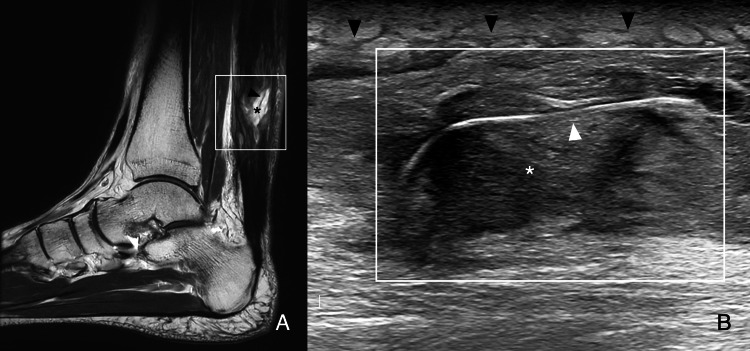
MRI and ultrasound (Patient 1) A) Sagittal T2-weighted image of the ankle shows a cystic-like lesion (black asterisk) aligned with the suture path (black arrowhead). B) Ultrasound B-mode, long axis: sutures (white arrowhead) embedded in heterogeneous material (white asterisk) with subcutaneous inflammatory changes (black arrowheads). Color Doppler also revealed hyperemia (not shown). The white box in A indicates the scanned region shown in B.
